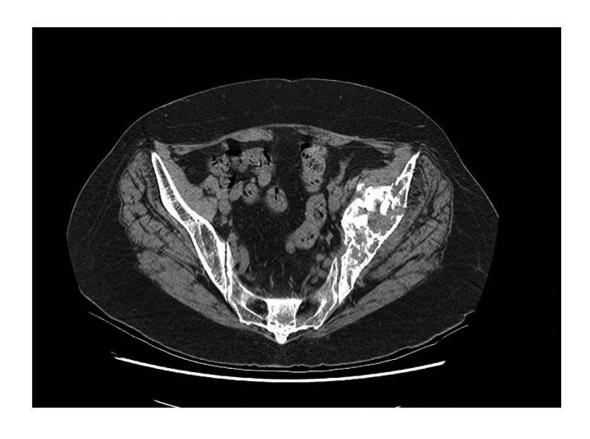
CT scan showing periacetabular bone loss.
